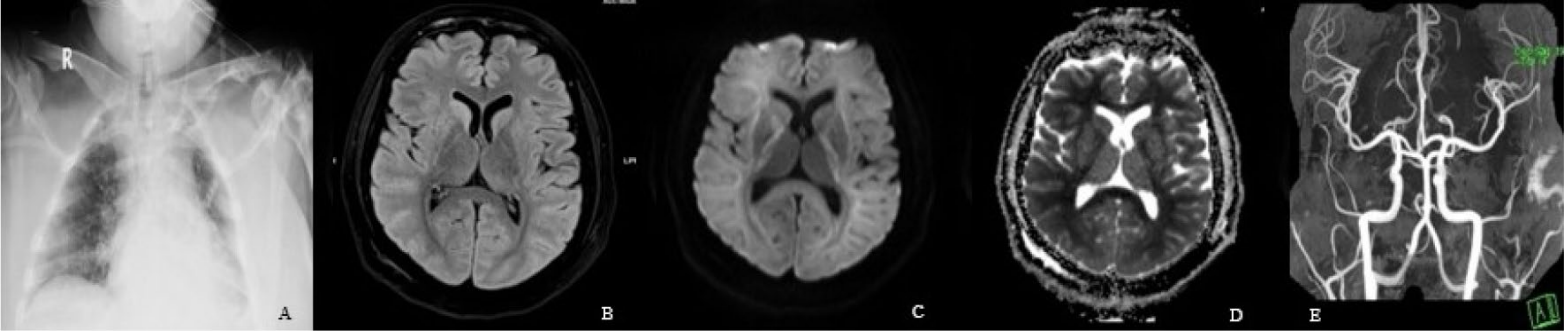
Twenty-six-year-old male with no prior co-morbidities; admitted with severe RT-PCR proved SARS-CoV-2 pneumonia, (A) as shown on the chest radiogram. This patient, who was on mechanical ventilation, went into cardiac arrest. Brain MRI was performed 4 days post arrest due to low GCS (= 6), revealed global hypoxic-ischemic encephalopathy. (B) Axial FLAIR image, at the level of the BG, showed decreased signal intensity in the thalami, while (C) corresponding DWI image showed lack of hyperintensity in both thalami, in addition to the caudate and putamen nuclei, findings that represent pseudo normalization. (D) Also, corresponding ADC map showed hyperintensity in the locations cited in (B). (E) Reconstructed maximum intensity projection magnetic resonance angiography (MRA) image shows no large vessel occlusion.
